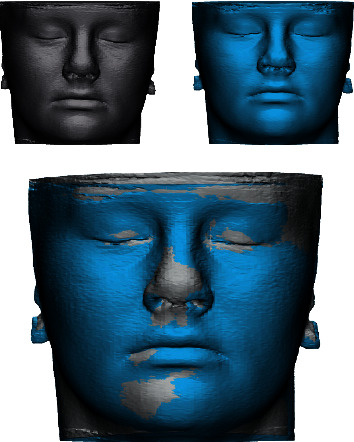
Example of surface-based superimposition of CBCT-derived patient's facial soft tissues before and after orthodontic treatment with maxillary expander.
